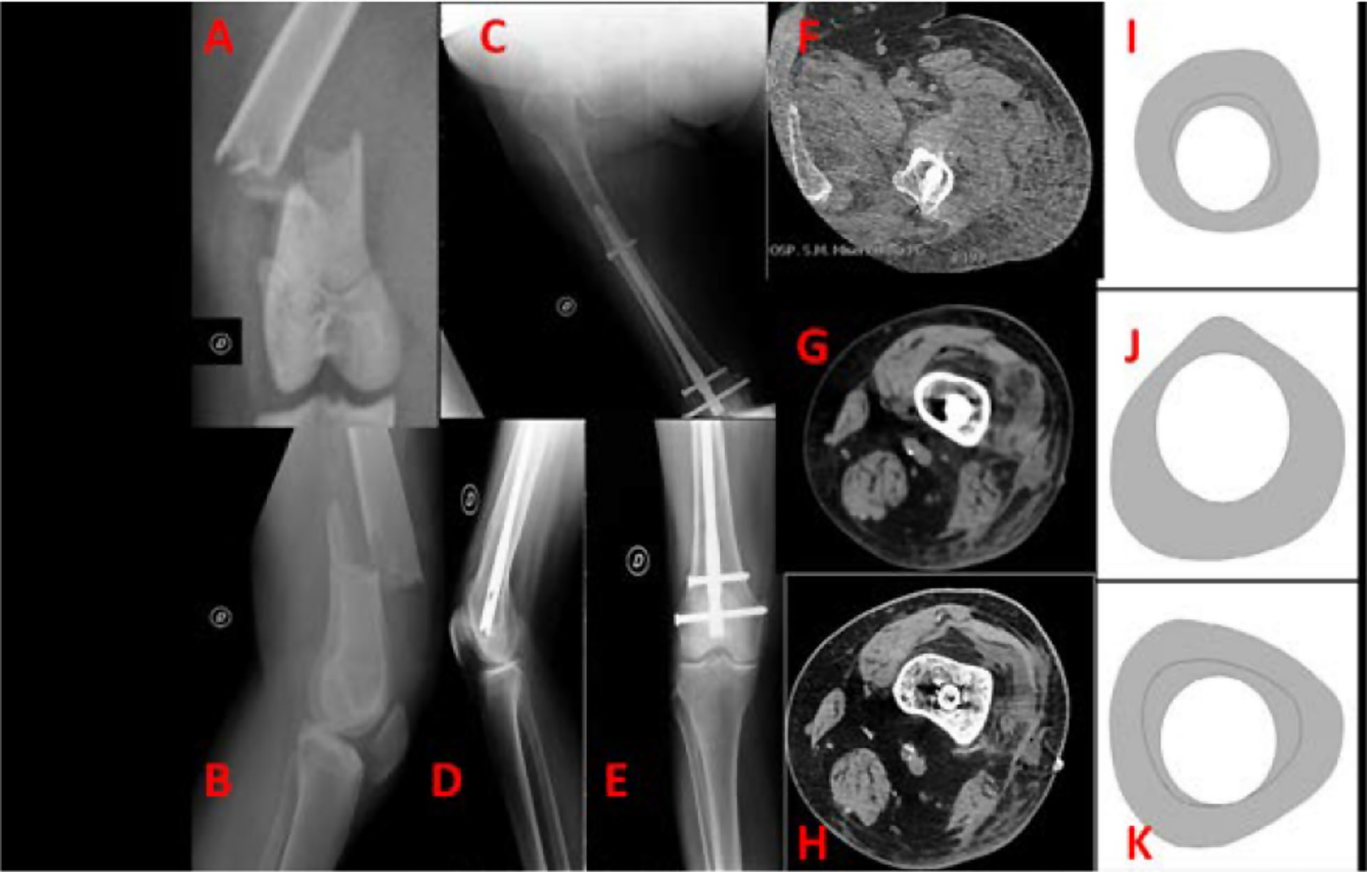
47F, road car accident, 32 A3 type of fracture (A, B). X-ray at 12 months post-operation (C–E) showed satisfactory fracture healing after locking retrograde nail. Absence of stress shielding of the nail is shown in F–H comparing images with biomechanics representations (I–K) proposed by Shih et al.
